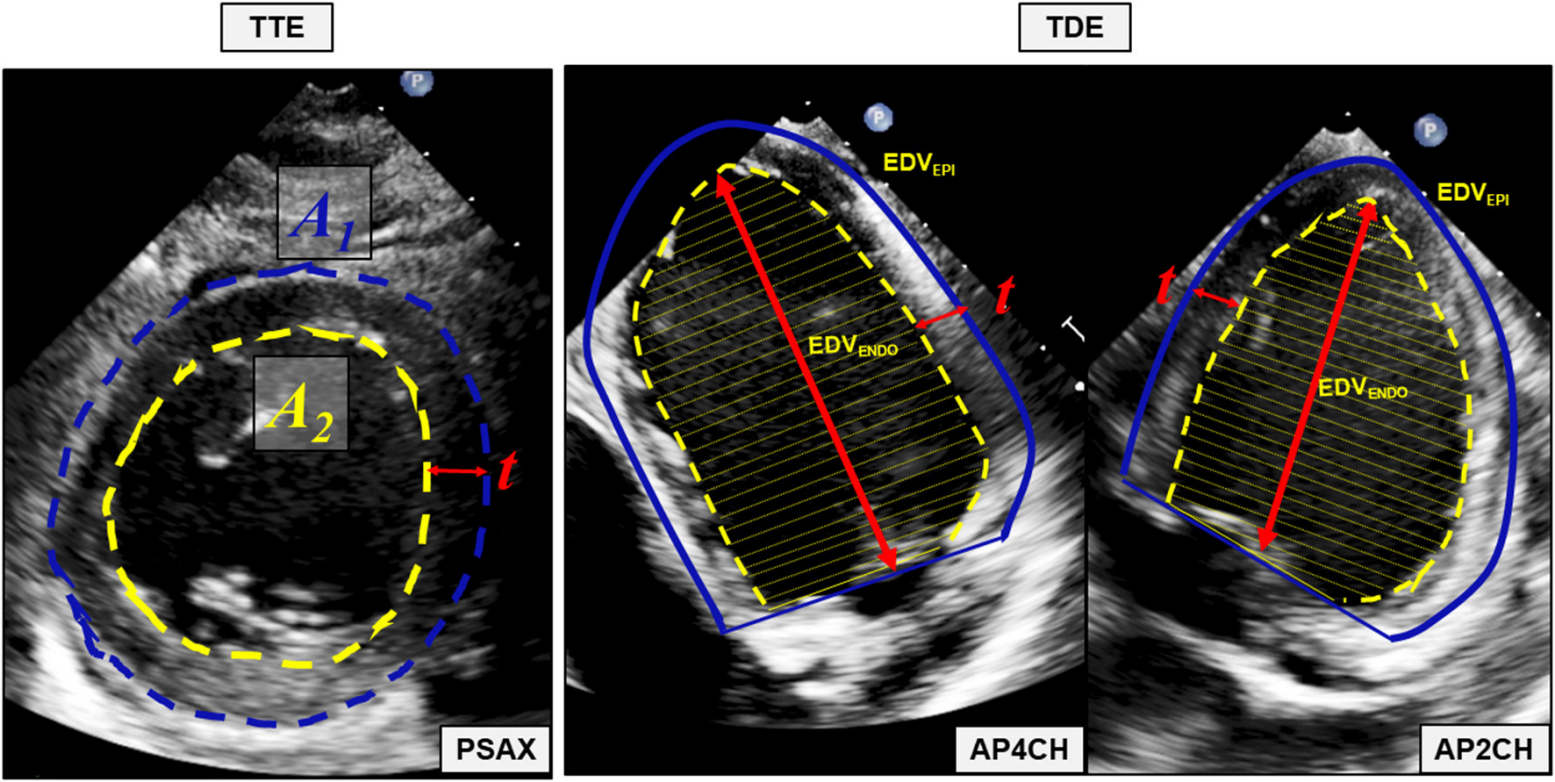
The novel 2D-based echocardiographic method for quantification of left ventricular mass. Mean wall thickness (*t*) is calculated by tracing of the endocardium (A_2_) and the epicardium (A_1_) in the parasternal short axis (PSAX) view. The left ventricular volume defined by the endocardium (EDV_ENDO_) is acquired using the biplane methods of disks with tracings of the endocardium in the apical four chamber (AP4CH) and apical two-chamber (AP2CH) view. Mean wall thickness (*t*) is added to each unique disk and a new volume, the left ventricular volume defined by the defined by the epicardium (EDV_EPI_) is quantified. Myocardial volume is calculated by subtracting EDV_ENDO_ from EDV_EPI_ and left ventricular mass is quantified by multiplying the myocardial volume with the myocardial density/gravity of 1.05 g/ml. TTE transthoracic echocardiography, TDE, transdiaphragmatic echocardiography; PSAX, parasternal short axis view; A_1_, Area defined by the epicardium; A_2_, Area defined by the endocardium; t, mean wall thickness; AP4CH, apical four chamber view; EDV_ENDO_, the left ventricular volume defined by the endocardium; EDV_EPI_, the left ventricular volume defined by the epicardium; AP2CH, apical two-chamber view.
